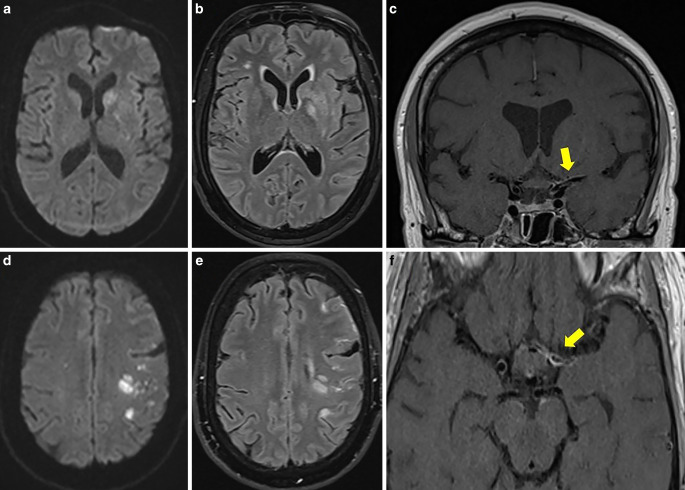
MRI examination after stenting of the left MCA in a patient with severe left M1 stenosis due to LNB-associated vasculitis. DWI images (b = 1000 s/mm2, axial views; a, d) and FLAIR images (axial views; b, e) demonstrated known ischemic lesions in the left basal ganglia and the left frontoparietal lobe, and residual sulcal SAH. Fat-saturated post-contrast T1-weighted images (coronal view—c; axial view—f) showing mural contrast enhancement in the left M1 segment and proximal A1 segment
